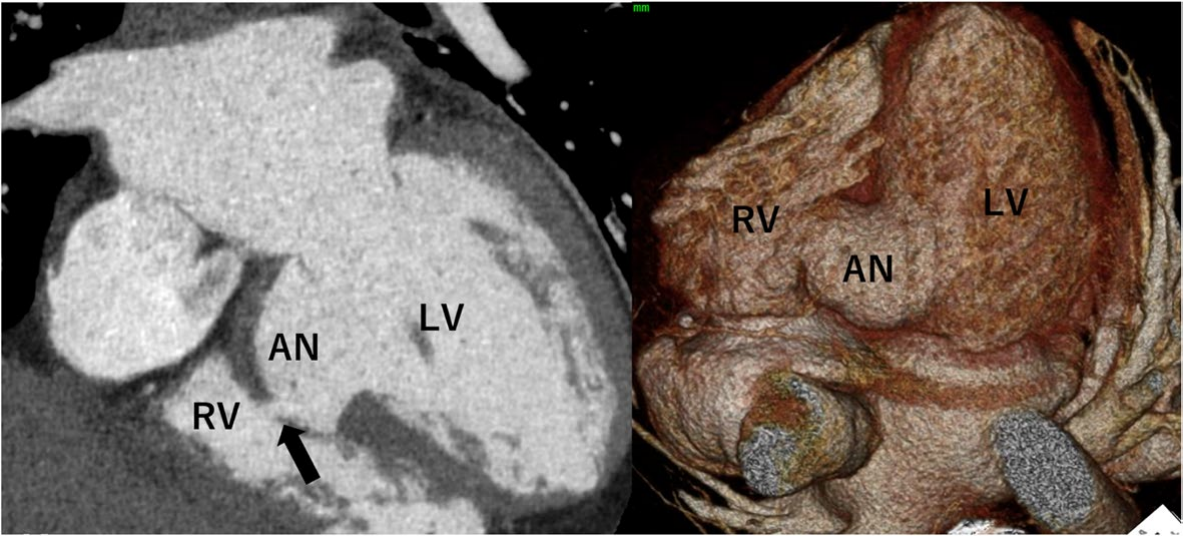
CT shows a 1 × 30 mm aneurysm (AN) on the LV posterior wall. The black arrow indicates perforation into the RV
